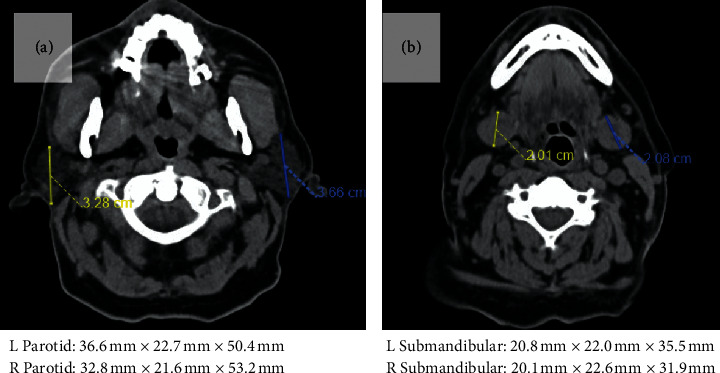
CT neck w/o contrast axial views demonstrating no enlargement of either the parotid gland (a) or submandibular gland (b).
